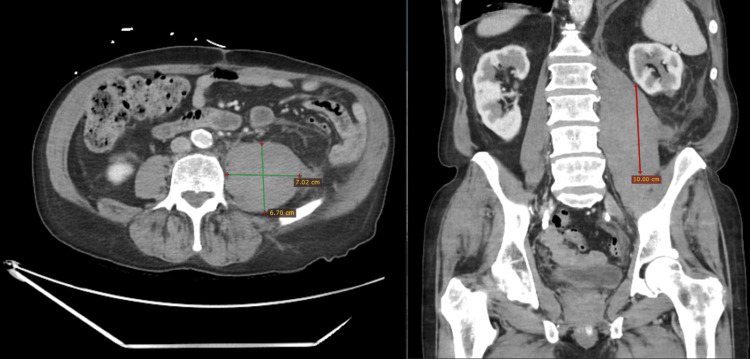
Contrast-enhanced CT of the abdomen and pelvis showing the largest cross-section of a left psoas intramuscular hematoma. Axial and coronal views demonstrate the hematoma measuring approximately 6.0 × 7.0 × 10.0 cm within the retroperitoneal space.
